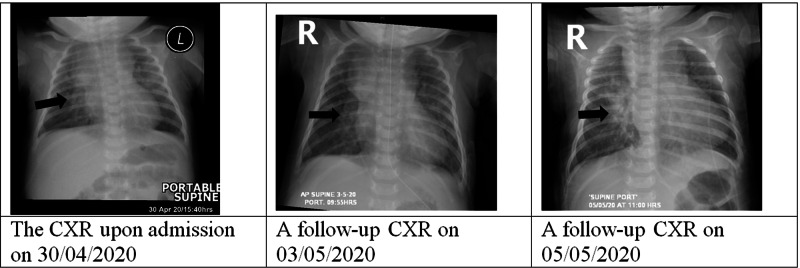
Serial chest X-ray (CXR) studies during the admission
